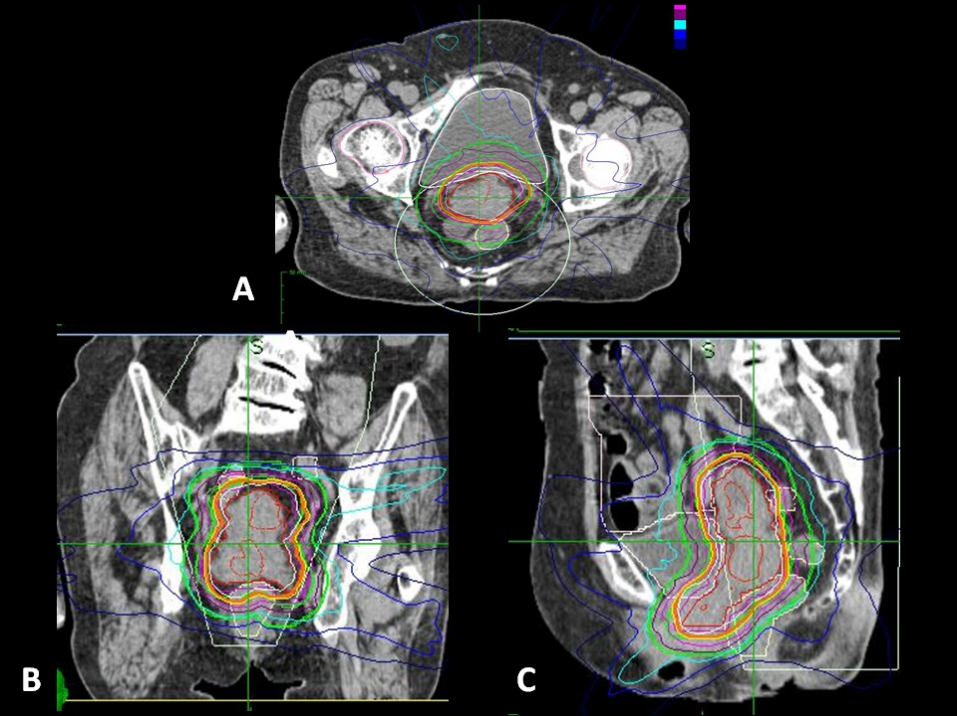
CyberKnife planning process of Case 1. Axial (A), coronal (B), and sagital (C) images of the planning CT. The tumor is contoured, and the volume and radiation doses are calculated accordingly. (CT: computed tomography)
